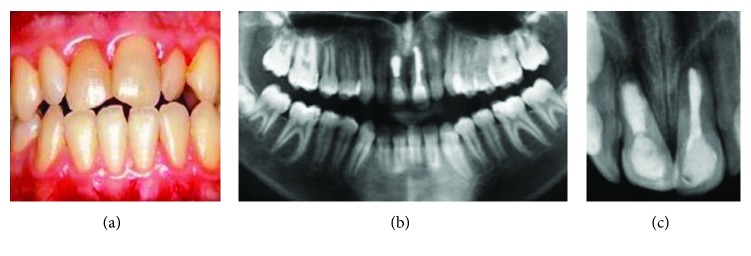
(a) Intraoral view of central incisors after 3 years. (b, c) Panoramic and periapical radiograph at 3-year follow-up.
